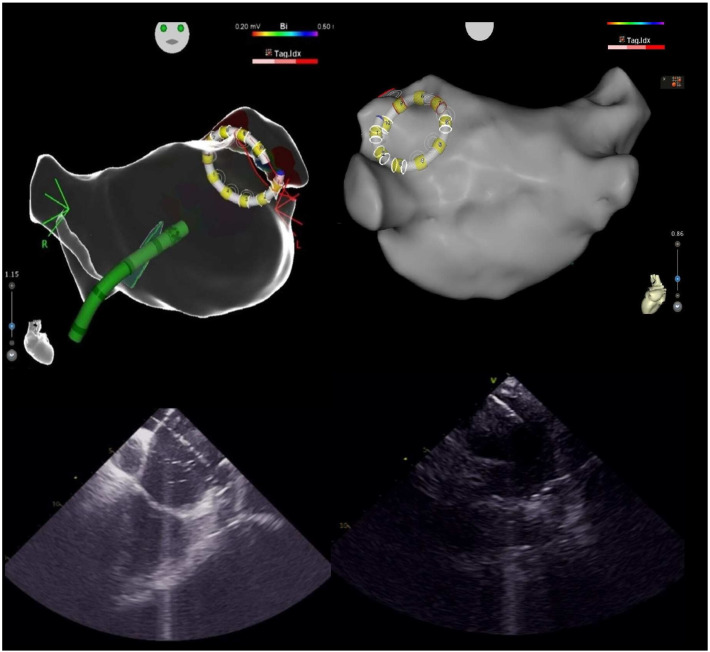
The top section shows map views reconstructed using the CARTOSOUNDFAM MAP Module with the VARIPULSE catheter introduced at the level of the left upper pulmonary vein, while the bottom section presents the ICE visualization of the same moment. The image on the right highlights the presence of a left common trunk with VARIPULSE catheter in ostial position, clearly visible also in the echocardiographic images.
